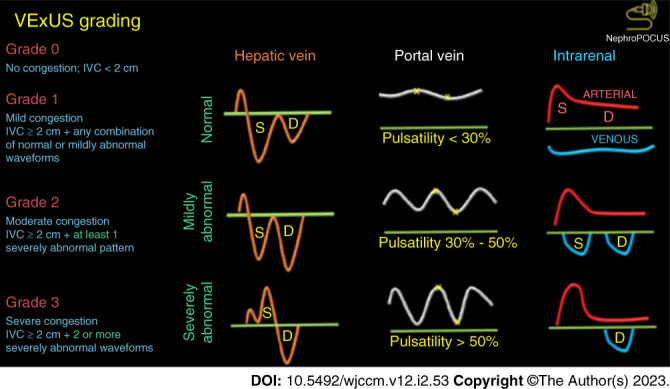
VExUS ultrasound depicting the relations between hepatic vein Doppler systolic (S) wave and diastolic (D) wave indicate varying degrees of congestion. Portal vein Doppler pulsatility similarly correlates with increasing congestion. Renal vein Doppler indicates increasing congestion when pulsatility becomes present in S and D waveforms. VExUS, venous excess ultrasound. Figure adapted from NephroPOCUS.com with permission.
